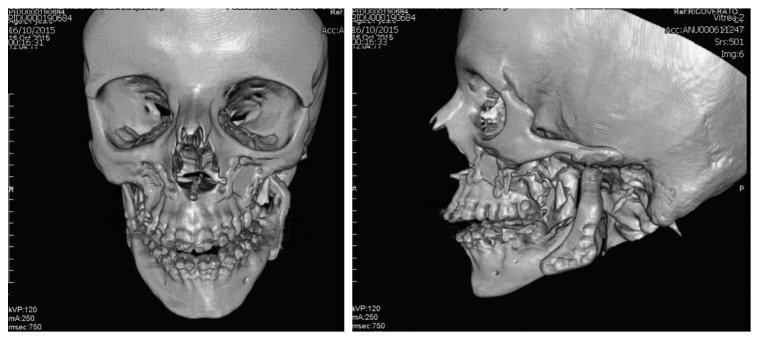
3D CT scan after surgery in frontal and lateral views.
